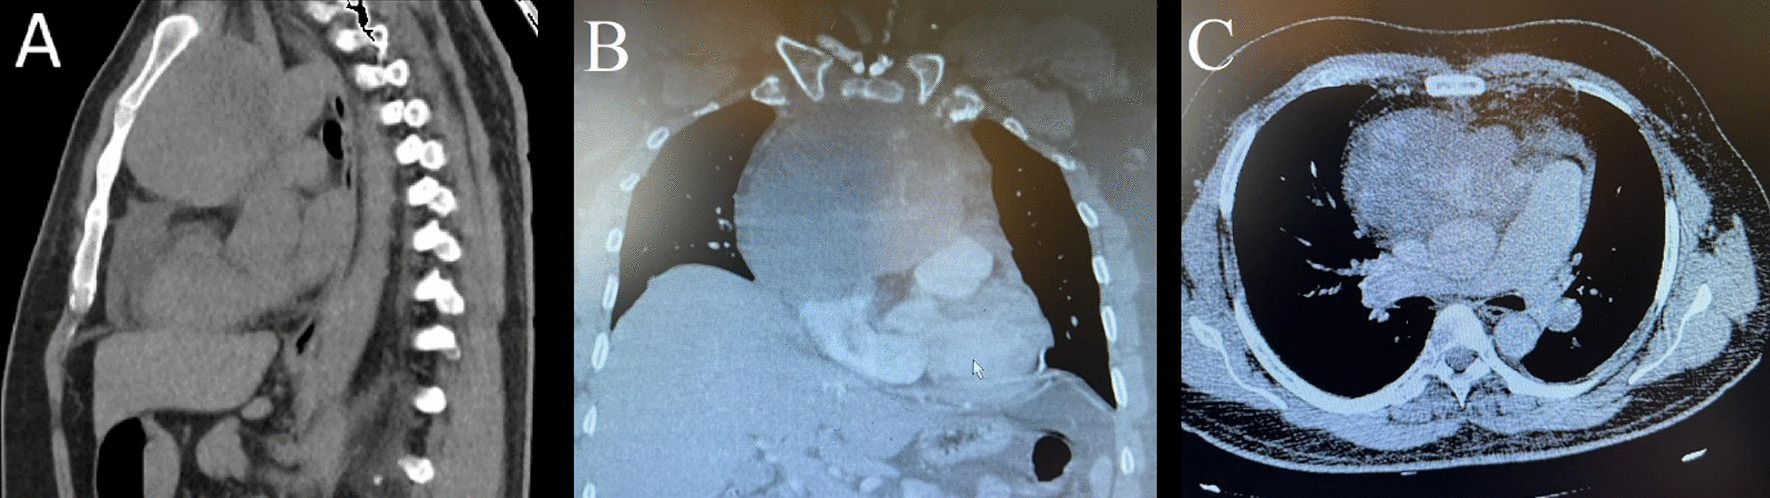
Chest computed tomography scan showing a large mediastinal mass; A Sagittal view, B coronal view, and C axial view
